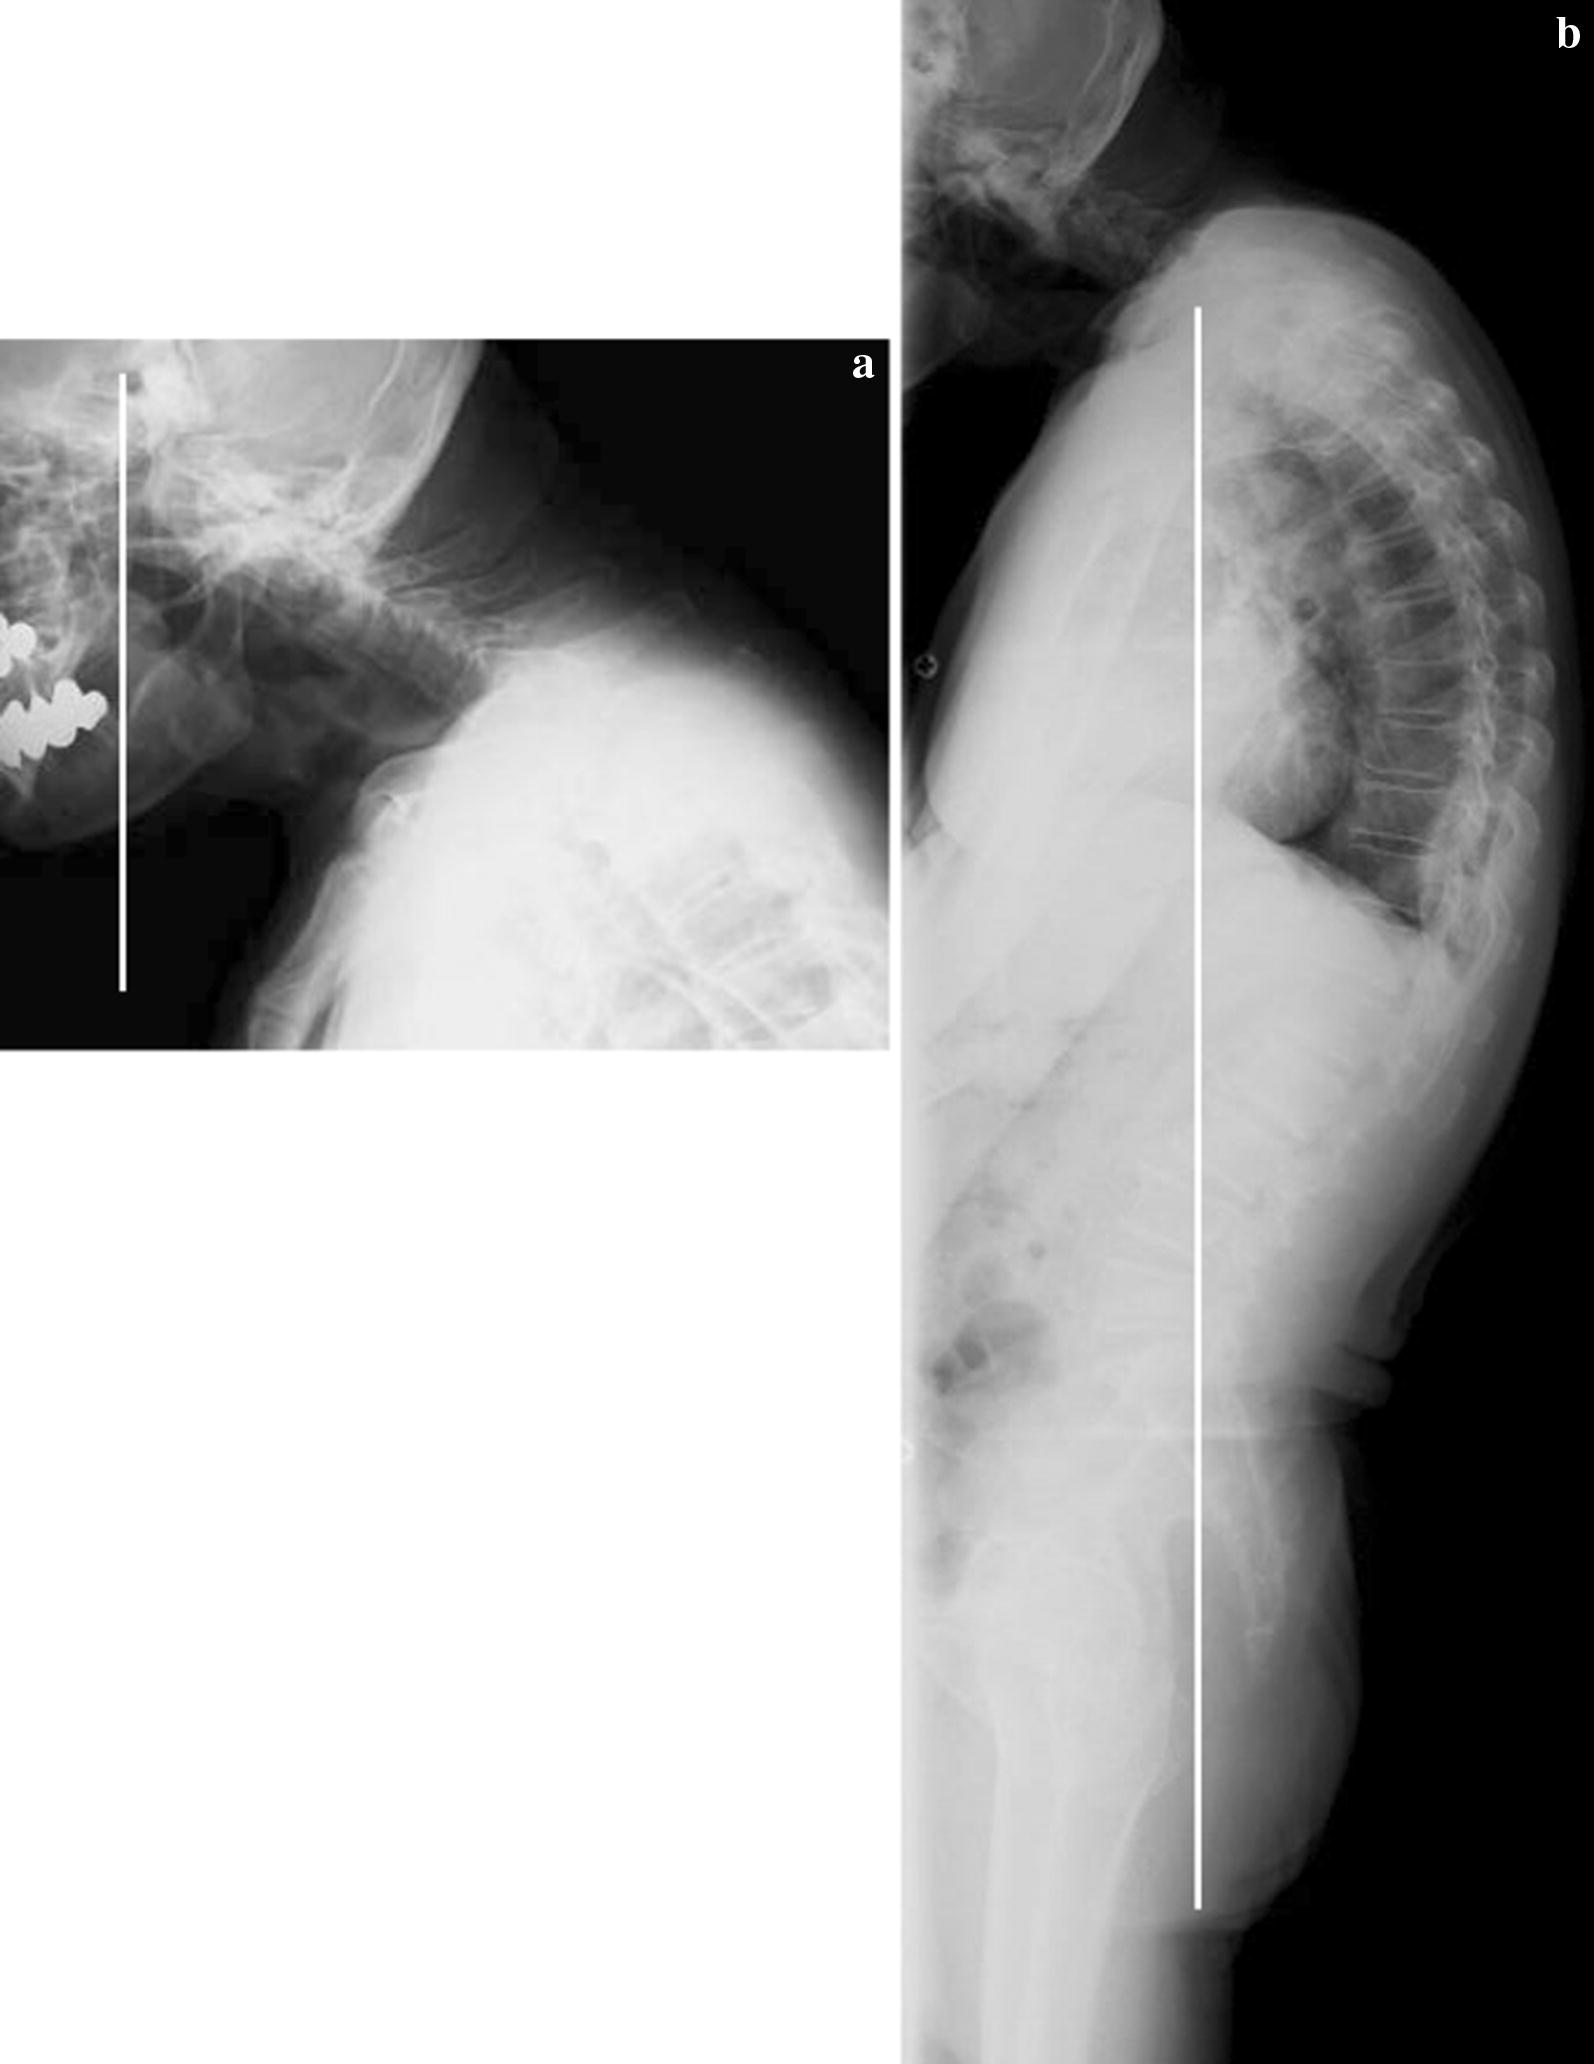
X-ray image before surgery. a Cervical X-ray lateral finding. White line is the center of gravity of the head-plumb line. b Whole spine X-ray lateral finding. White line is the C7-plumb line
